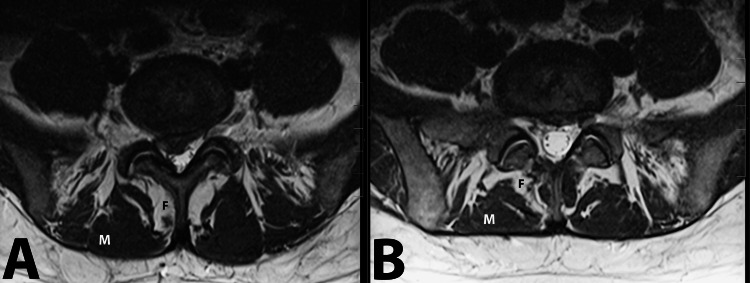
Axial T2-weighted MRI images of Patient 1 showing the spine at initial presentation (A) and one year after endoscopic discectomy (B). Along with the successful decompression of the L5-S1 space, please note the significant decrease in the multifidus muscle fatty infiltration (black letter F) and its atrophy (white letter M).
